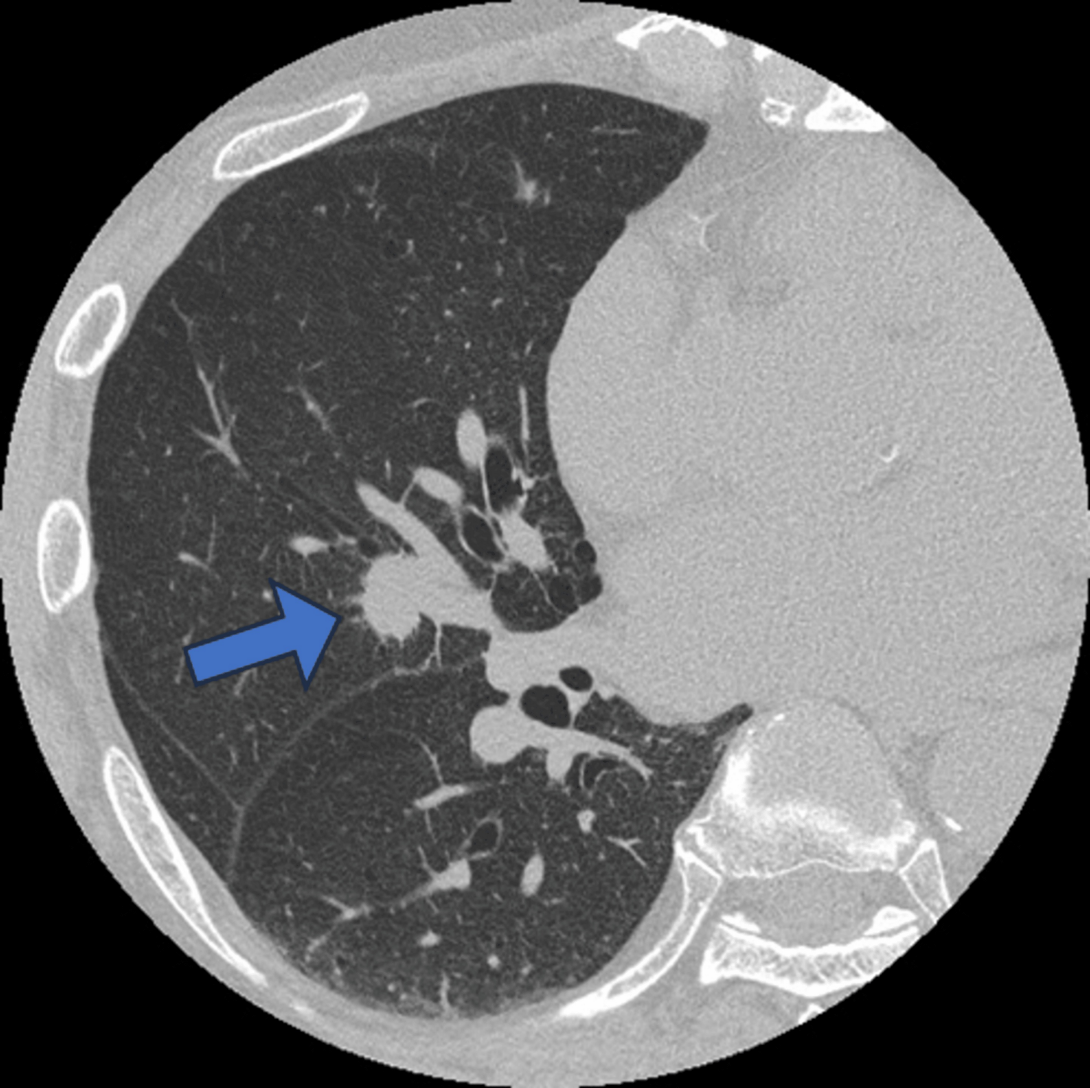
Preoperative chest computed tomography The axial image shows a solid nodule in the right middle lobe of the lung (arrow). This lesion was confirmed to be an adenocarcinoma using bronchoscopic biopsy.
